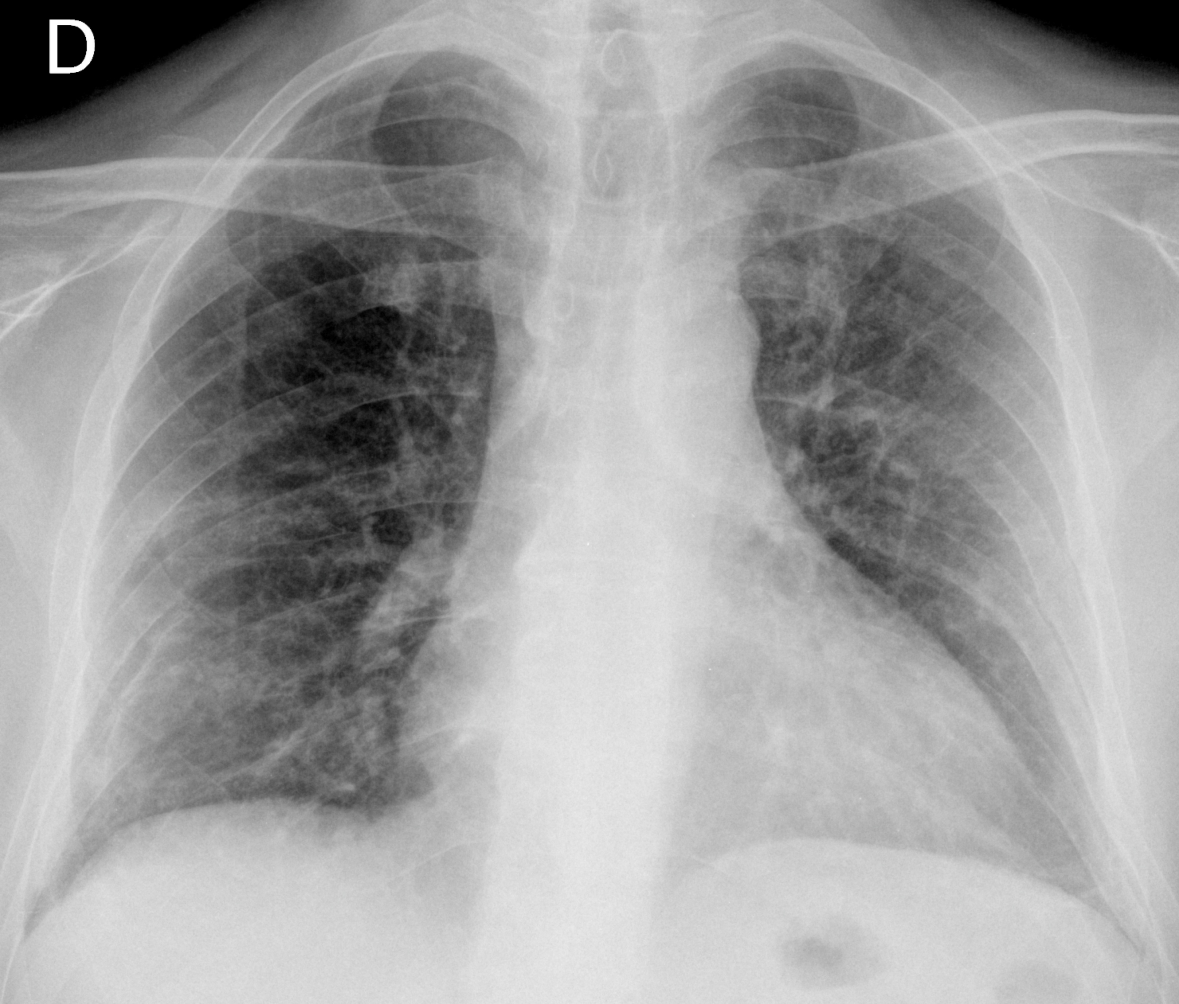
Chest x-ray after one month of steroid treatment
